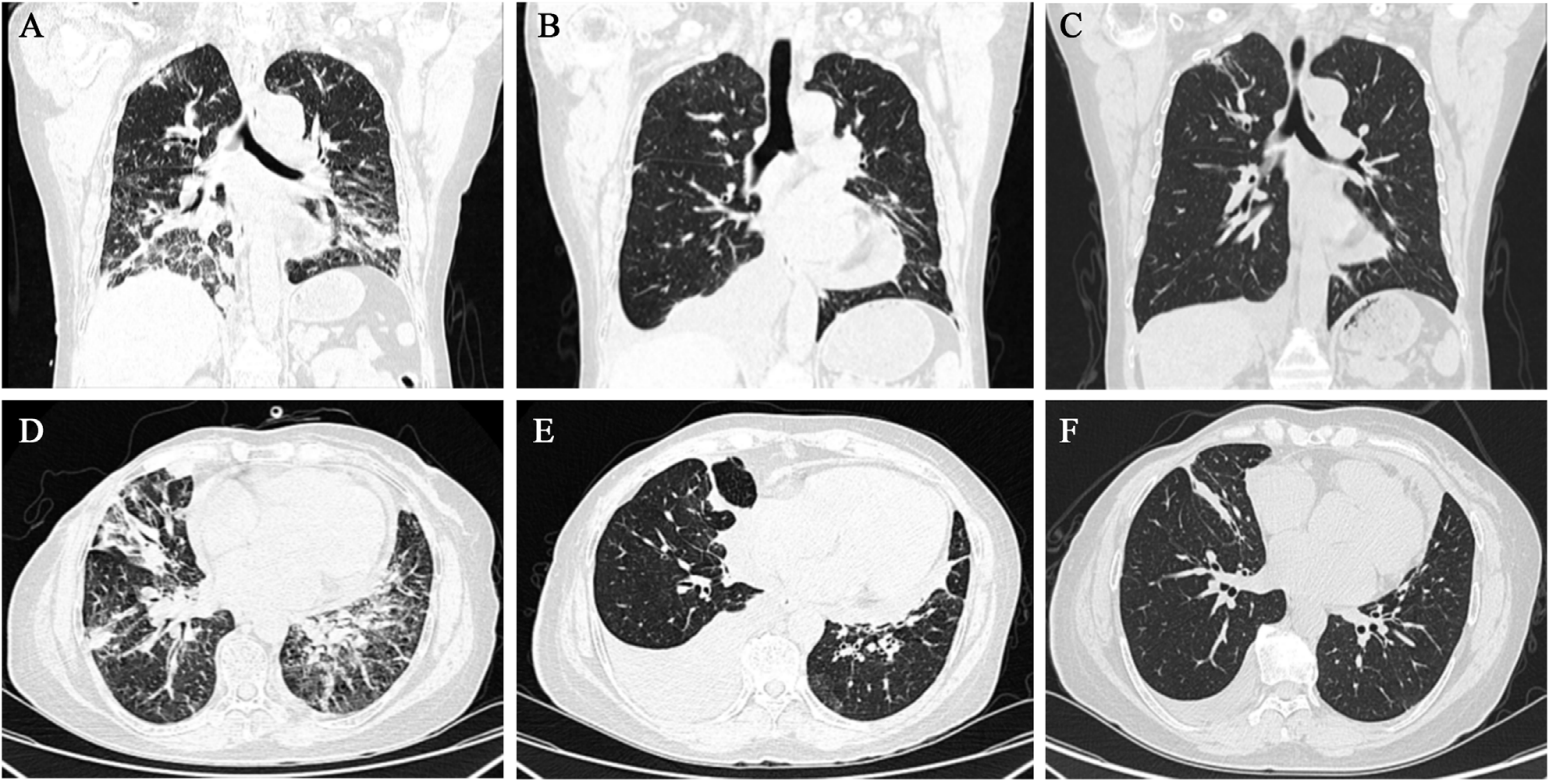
Chest CT showed gradual improvement of PLC following T-DXd treatment. (A–C) Cross-sectional images taken on 20 March 2023, 10 July 2023, and 14 November 2023, respectively. (D–F) Coronal images taken on 20 March 2023, 10 July 2023, and 14 November 2023, respectively.
